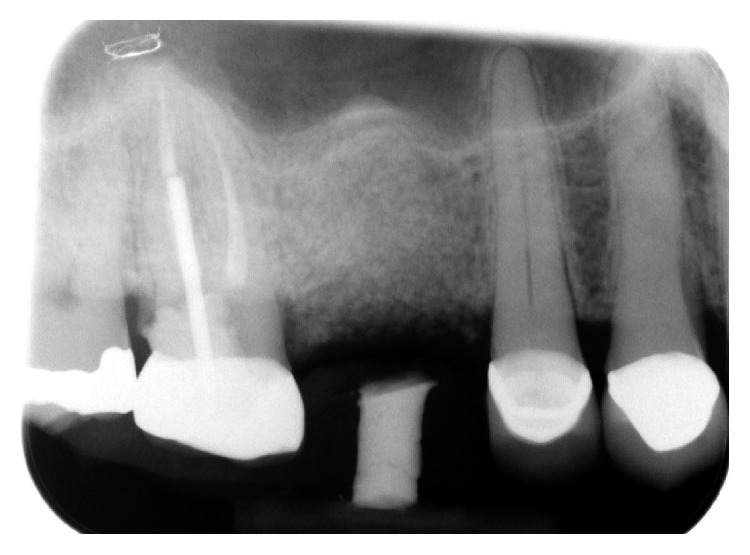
A periapical radiograph 3 months after the extraction and ridge preservation showed no signs of residual pathology.
